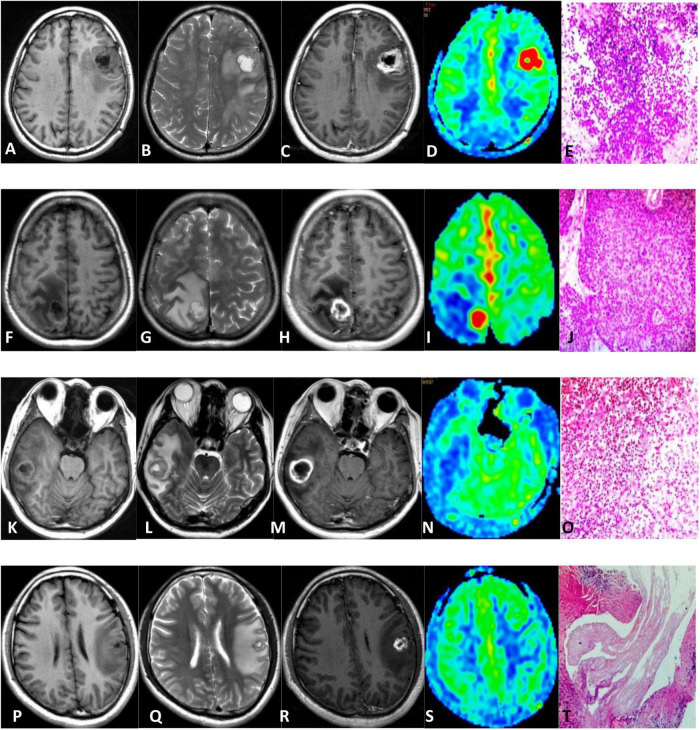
Comparison of HGG (A–E), metastasis (F–J), brain abscess (K–O), and cerebral cysticercosis (P–T). First row: 64-year-old male with an HGG in the left frontal lobe; unenhanced MR scan showed hypointense on T1WI and hyperintense onT2WI (A,B). The contrast-enhanced T1-weighted MRI showed ring enhancement (C). 3D-pCASL exhibited as hyper-perfusion in the region of tumor compared with the contralateral brain (D). Pathologically, the tumor cells were diffusely distributed and showed increased density (E). Second row: 42-year-old woman with a metastatic tumor in the right parietal lobe; MR plain scan showed hypointense on T1WI and hyperintense on T2WI (F,G). The enhanced scan showed ring enhancement (H). 3D-pCASL showed as hyper-perfusion in the region of metastasis (I). Pathology showed the nested atypical cells, which was squamous cell carcinoma (J). Third row: 40-year-old man with a brain abscess in the right temporal lobe. MR plain scan showed hypointense on T1WI and hyperintense on T2WI (K,L). The enhanced scan showed ring enhancement (M). 3D-pCASL showed the lesion demonstrated as hypo-perfusion (N). Pathology showed lymphocyte, neutrophil infiltration, abscess formation, and granulation tissue hyperplasia (O). Fourth row: 28-year-old man with cerebral cysticercosis in the left frontal and parietal lobe. MR plain scan showed hypointense on T1WI and hyperintense on T2WI (P,Q). The enhanced scan showed ring enhancement (R). 3D-pCASL showed that the lesion was iso-hypo-perfusion (S). Pathologically, lace-like parasites can be seen (T).
